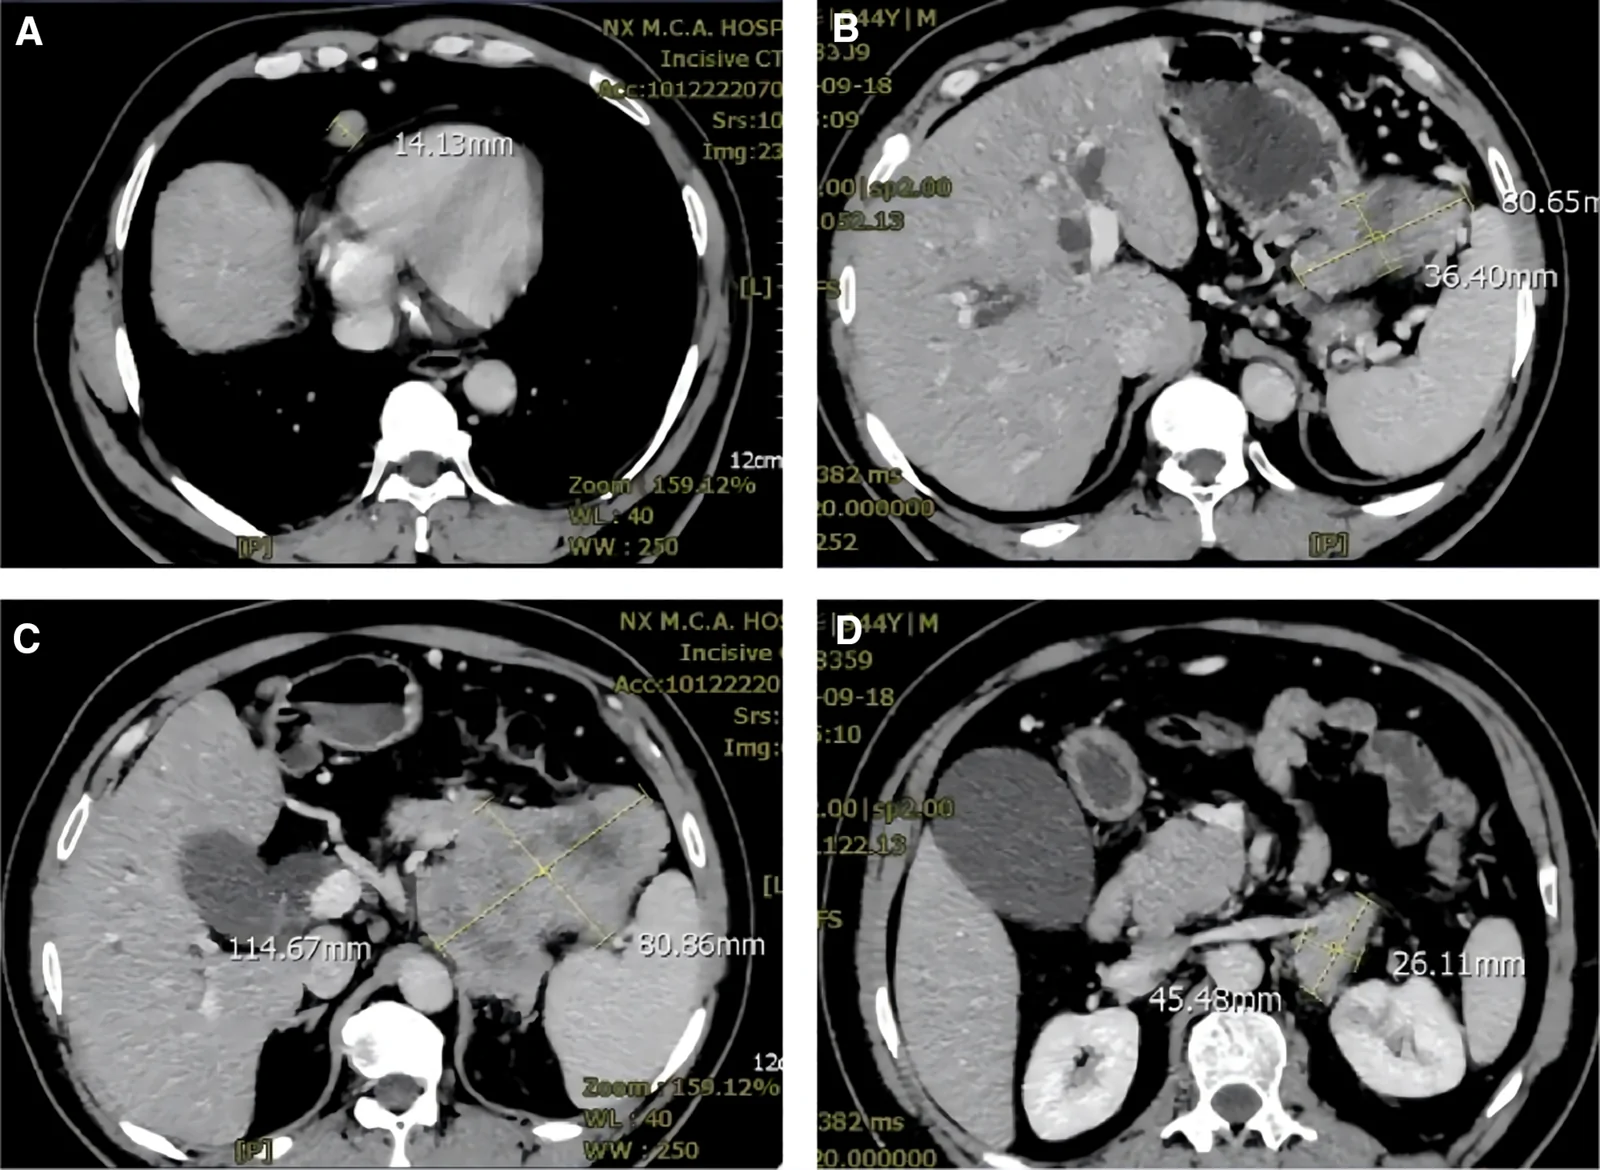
Contrast-enhanced CT of the chest and abdomen (September 2024). (A) Mediastinal lymphadenopathy. (B) Pancreatic mass. (C, D) Multiple large intra-abdominal masses. CT = computed tomography.
